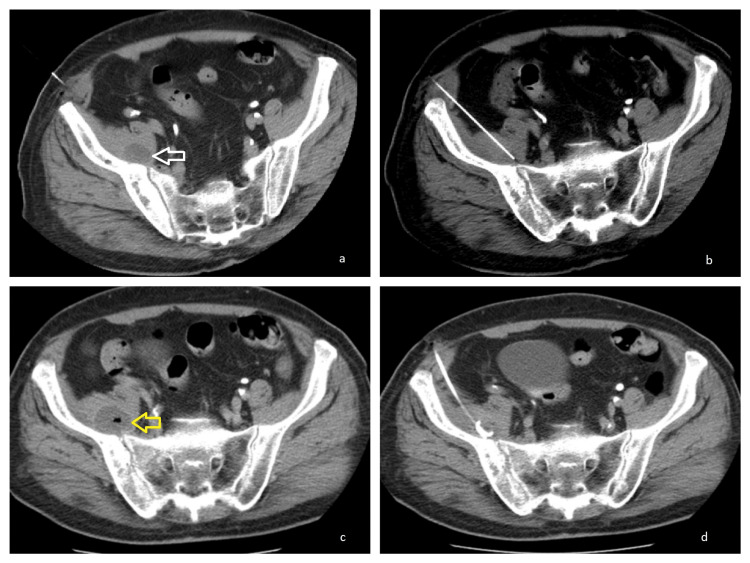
Computed tomography (CT) of the pelvis after intravenous contrast administration a and b: Guided paracentesis of the inflammatory fluid collection of the right iliac muscle (white arrow). c and d: Increase of the size of the inflammatory fluid collections of the right iliac muscle adjacent to the right iliac bone and the right sacral wing, currently measuring 3.6 x 2.5 x 6 cm, with increased density inside the cavity suggesting liquid rich in protein or hemorrhagic content, accompanied by peripheral enhancement (yellow arrow). Second CT-guided drainage of the iliac muscle fluid collection – anterior approach.
